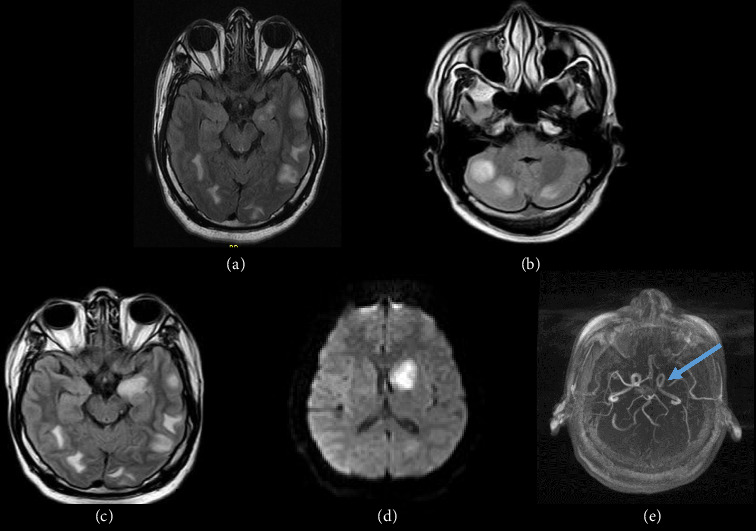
Brain MRI/MRA findings in a CNS tuberculosis patient with paradoxical response. (a, b) Baseline FLAIR: multiple supra- and infratentorial cortico-subcortical tuberculomas. (c) Post 2 month treatment: known tuberculomas with increased left basal ganglia edema. (d) DWI at 2 months: new ischemic lesion in left basal ganglia. (e) MRA: stenosis of left M1 and A1 segments (arrow).
